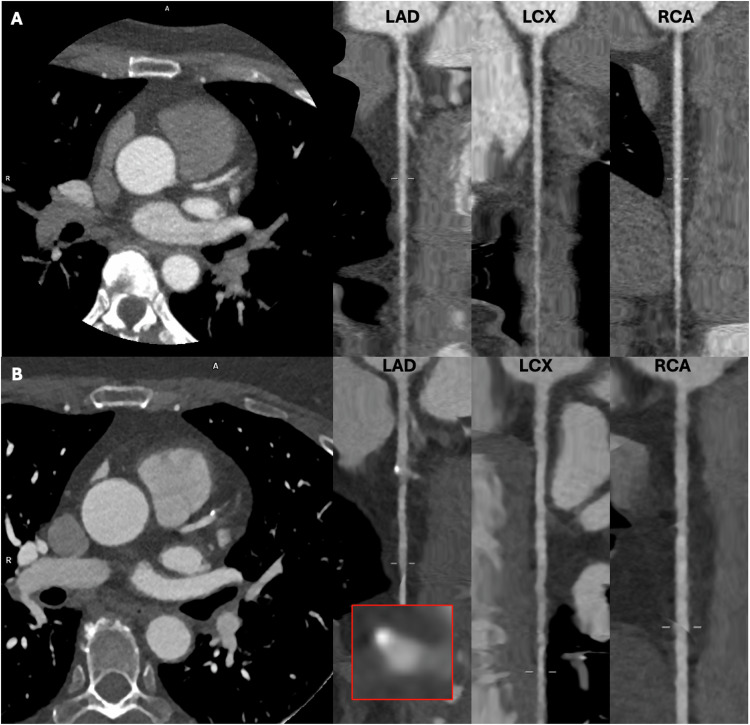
Development of CAD in a female patient, age 51 years, with a body mass index of 31 kg/m^2^, typical chest pain, and a family history of CAD. Baseline coronary CT angiogram (A) with axial image and curved planar reformations of the left anterior descending (LAD), left circumflex (LCx), and right coronary arteries (RCA) were normal. Coronary CT angiogram (B) 11 years later showed a calcified plaque in the mid LAD, demonstrated on the axial image, curved planar reformation, and a cross-sectional image (red box)
